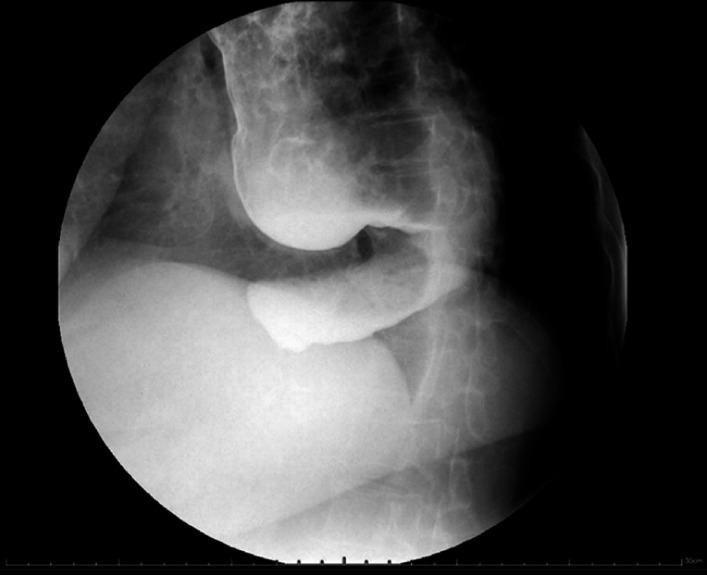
Radiographic image showing the sigmoid esophagus.
